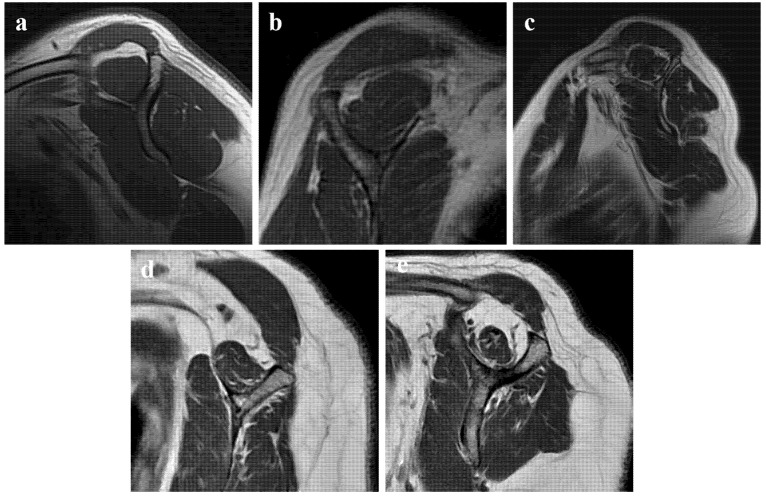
Goutallier grading of supraspinatus fatty infiltration (FI) on sagittal MRI. (a) Grade 0: normal muscle without fatty streaks (no FI). (b) Grade 1: slight FI manifested as a few fatty streaks within predominantly muscular tissue. (c) Grade 2: more muscle than fat; fatty areas are clearly visible but muscle still predominates. (d) Grade 3: approximately equal proportions of muscle and fat. (e) Grade 4: more fat than muscle, with severe FI and marked loss of contractile tissue.
